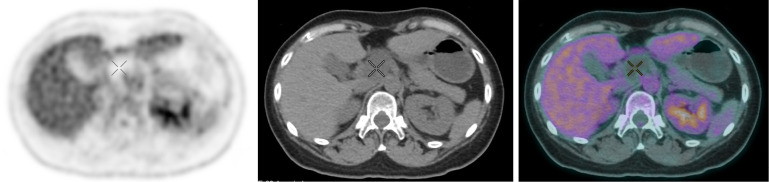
FDG PET (left) showed hypometabolism in the concerned lesion abutting the caudate lobe of liver (cross).
